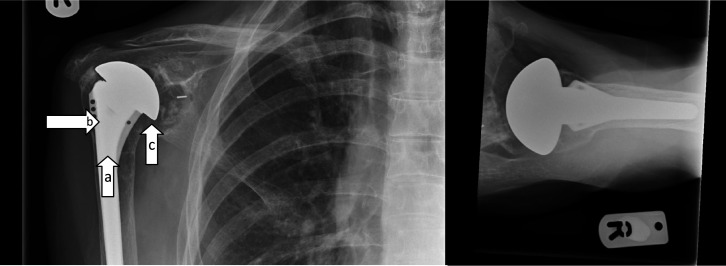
X-rays taken at initial consultation demonstrating elevation of the humeral head (a), medialization of the glenoid implant (b) and osteolysis around the humeral implant (c).
